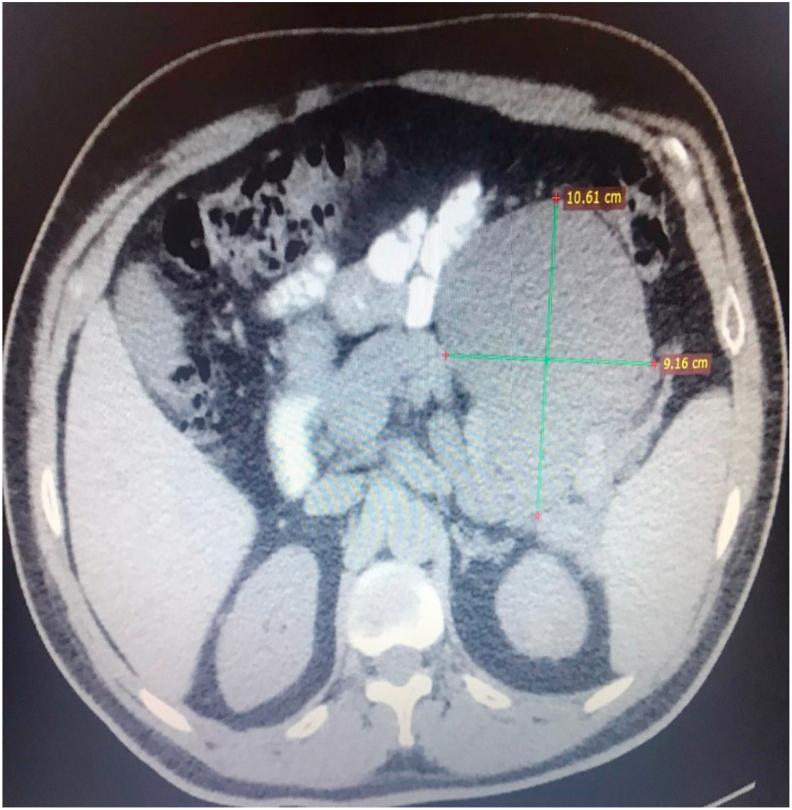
Abdominal CT scan showing a huge pancreatic pseudocyst occupying the body and tail of the pancreas.
